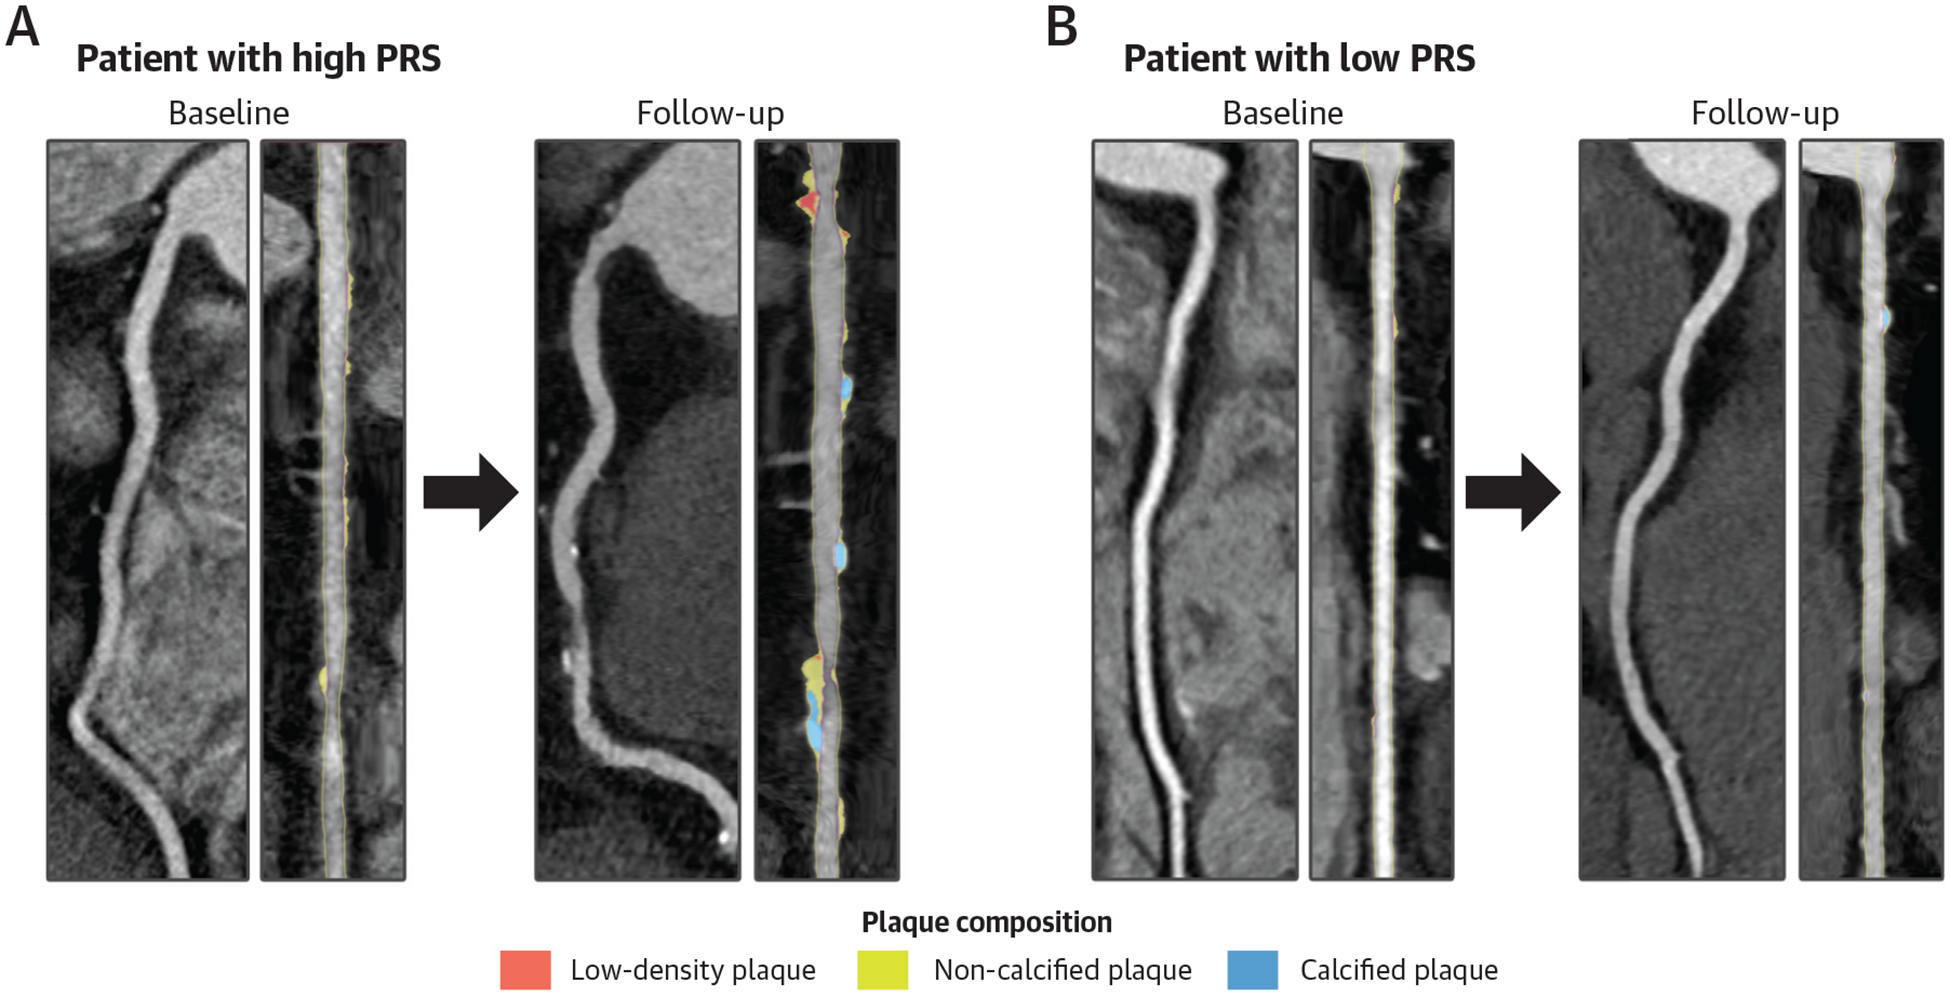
Coronary Plaque Progression and HRP in Patients With High and Low Polygenic Risk Shown are 2 case examples of a patient with high (A) and low (B) PRS. Shown are baseline coronary CTA curved reformatted (left) and straightened multiplanar reformatted (right) reconstructions of the right coronary artery. At baseline, patient A (high CAD PRS, 98th percentile) had a PAV of 16.5%, a percent noncalcified plaque volume (NCPV) of 6.4%, and a percent calcified plaque volume (CPV) of 10.0%, with no presence of HRP. During the 10-year follow-up, the plaque volumes for PAV, percent NCPV, and percent CPV progressed to 26.1%, 11.5%, and 14.6% at follow-up (58% PAV increase, 80% percent NCPV increase, and 46% percent CPV increase), respectively, indicative of important plaque progression. Furthermore, 2 HRPs with low-density plaque had developed. Patient B (low CAD PRS, 10th percentile) had a baseline PAV of 5.8%, a percent NCPV of 4.5%, and a percent CPV of 1.3%. During the 10-year follow-up, the plaque volumes for PAV, NCPV, and CPV changed to 7.6%, 4.9%, and 2.5% (31% PAV increase, 8% NCPV decrease, and 92% CPV increase), indicative of relative plaque stabilization. No HRPs developed throughout the follow-up. Abbreviations as in Figure 1.
